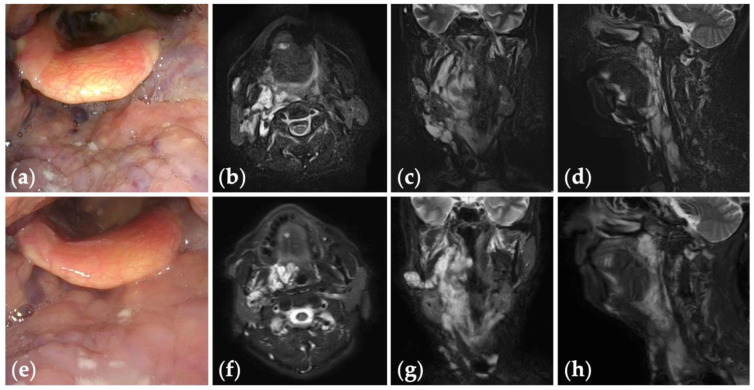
Patient 2 graded “good” for treatment outcomes after seven sessions of fluoroscopy- and endoscopy-guided transoral sclerotherapy: (a) an initial endoscopic photograph of the pharynx of a 71-year-old man with oropharyngolaryngeal venous malformation, and (e) the latest endoscopic photograph six months after the fifth sclerotherapy session at the age of 73 years; (b–d) short tau inversion recovery T2-weighted magnetic resonance images before treatment, and (f–h) three years after the final sclerotherapy session at the age of 78 years.
